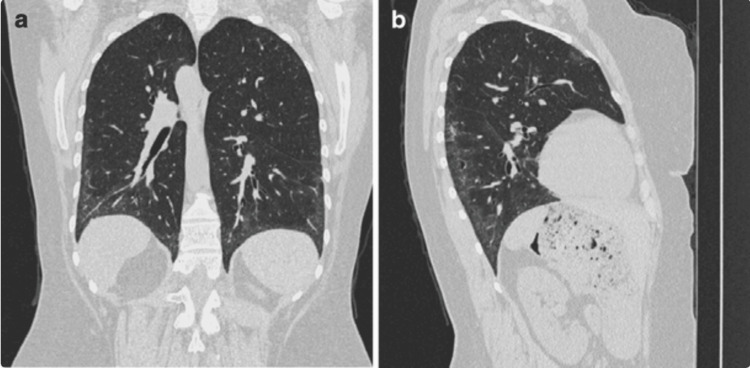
Early radiological features of SSc-ILD on chest CT. Coronal and sagittal chest CT reconstructions demonstrate the classic appearance of early lung involvement in systemic sclerosis-associated interstitial lung disease (SSc-ILD), with subtle ground-glass opacities and reticular changes predominantly in the peripheral, posterior, and basal lung regions. Reproduced from Strollo and Goldin (2010) [10]. Image reproduced from an open-access article distributed under the terms of the Creative Commons Attribution Noncommercial License.
